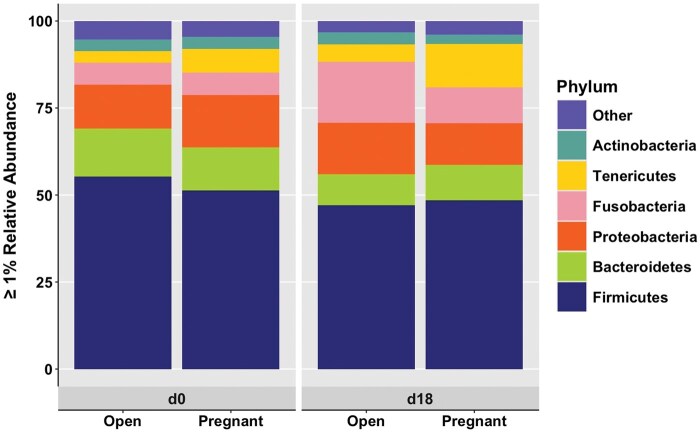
Phyla greater than 1% relative abundance between days (d0 and d18) and pregnancy status (Open and Pregnant). Relative abundance as a percent is shown on the y-axis with pregnancy status within days shown on the x-axis. Differing colors represent different phyla found in greater than 1% relative abundance within the vagina. Phyla less than 1% relative abundance are combined into the “Other” category. Pregnancy status was determined by transrectal ultrasonography on d32 post-artificial insemination.
